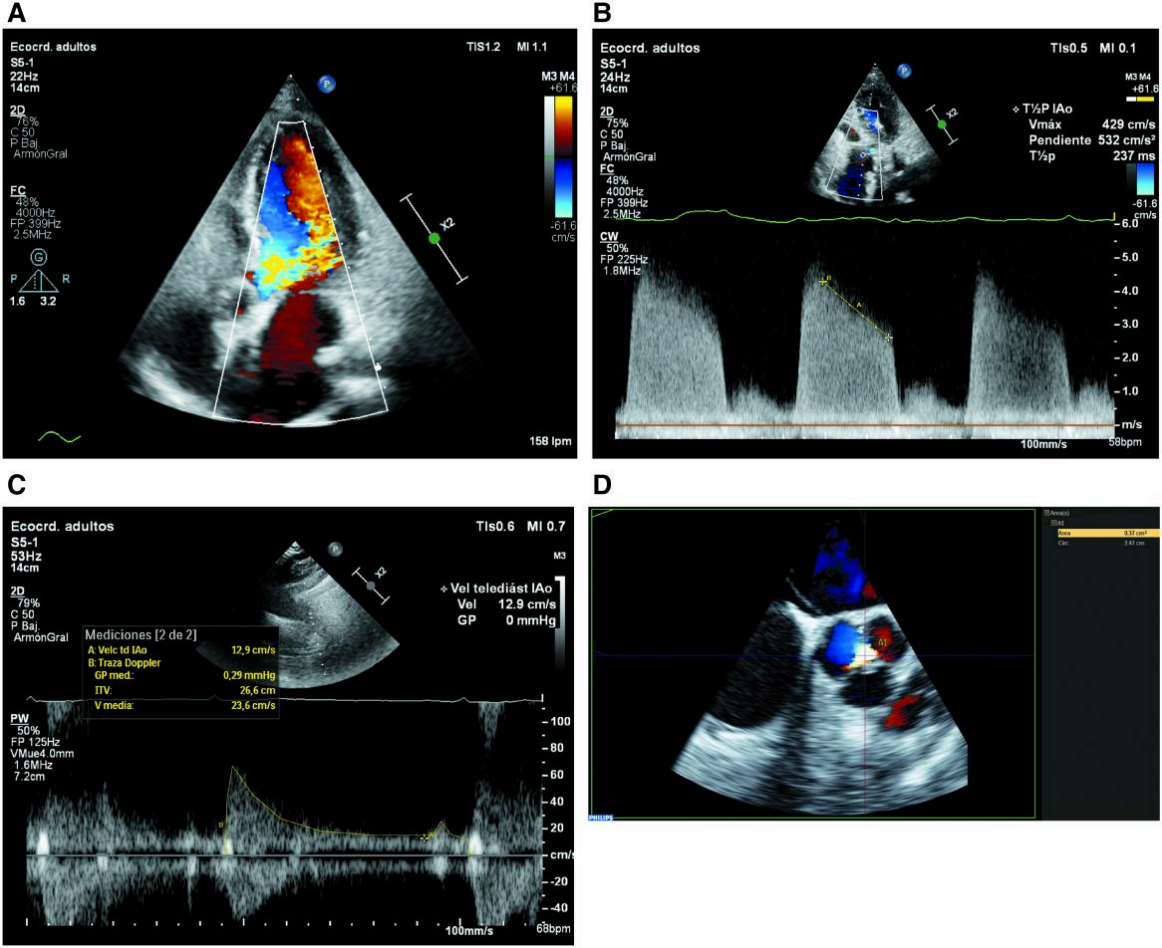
Severe aortic regurgitation. (*A*) Doppler showing the aortic regurgitation jet. (*B*) Continuous-wave Doppler showing a steep slope of the regurgitant jet (PHT < 250 ms). (*C*) Pulsed Doppler with VTI measurement in the descending thoracic aorta (VTI: 26 cm). (*D*) Multiplanar planimetric measurement of the effective regurgitant orifice area (EROA: 37 mm²).
